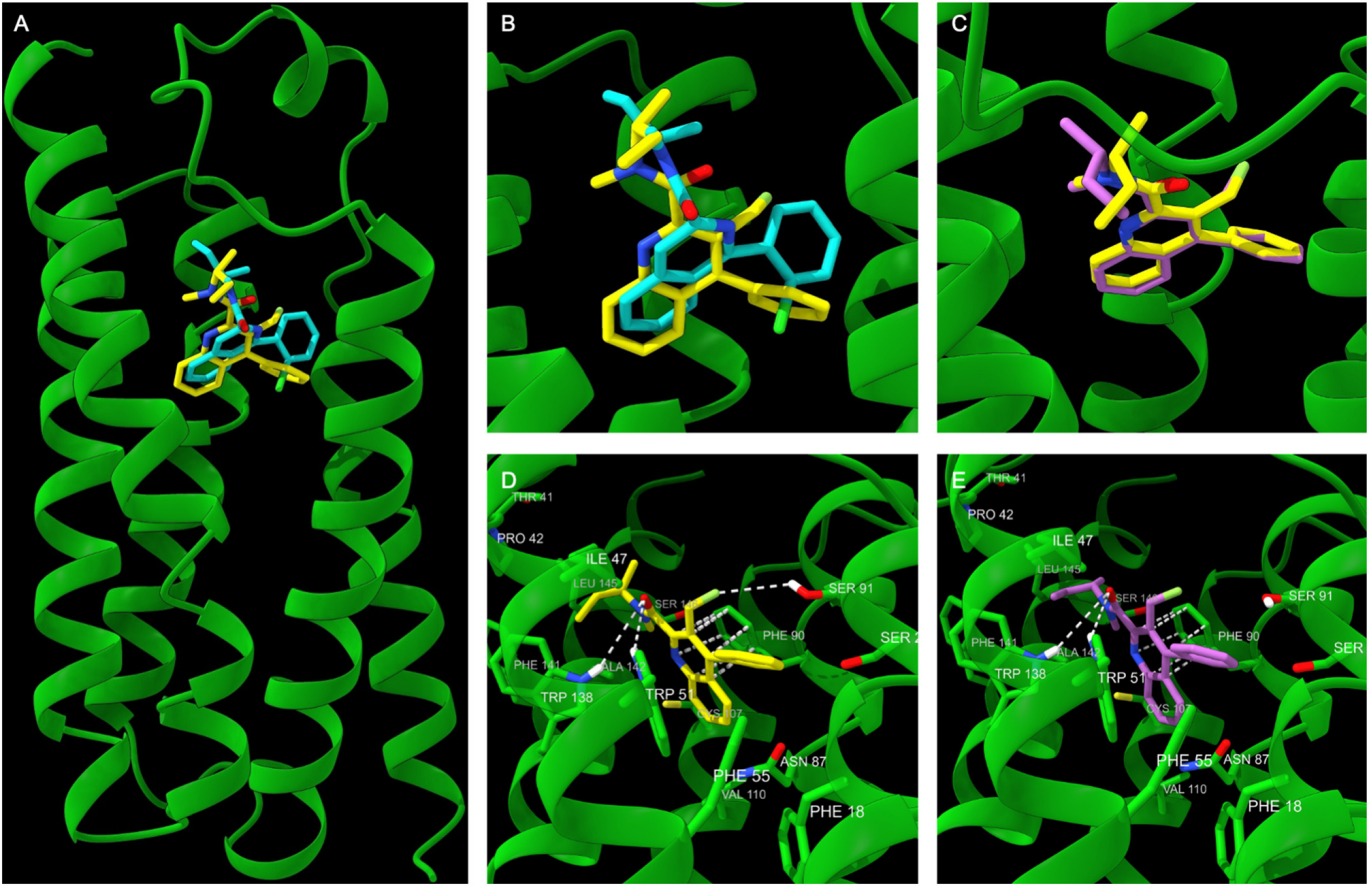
Docking studies with the X-ray structure of *Bacillus cereus* TSPO. A) Superimposed (*R*)-LW223 (yellow) and PK11195 (cyan). B) Close up view of superimposed (*R*)-LW223 (yellow) and PK11195 (cyan). C) Close up view of superimposed (*R*)-LW223 (yellow) and (*S*)-LW223 (violet). D) Close up view of docked (*R*)-LW223 showing key interactions. E) Close up view of docked (*S*)-LW223 showing key interactions.
